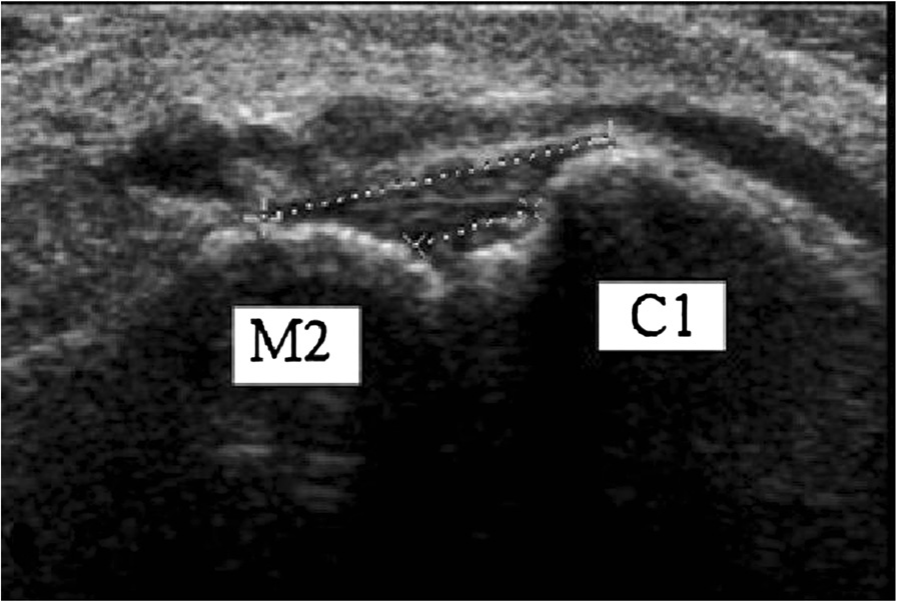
Close-up sonographic image of the dorsal Lisfranc ligament. C1 = Medial cuneiform, M2 = 2^nd^ metatarsal base, Dashed line with cross hairs indicate the boundaries of the dorsal Lisfranc ligament.
